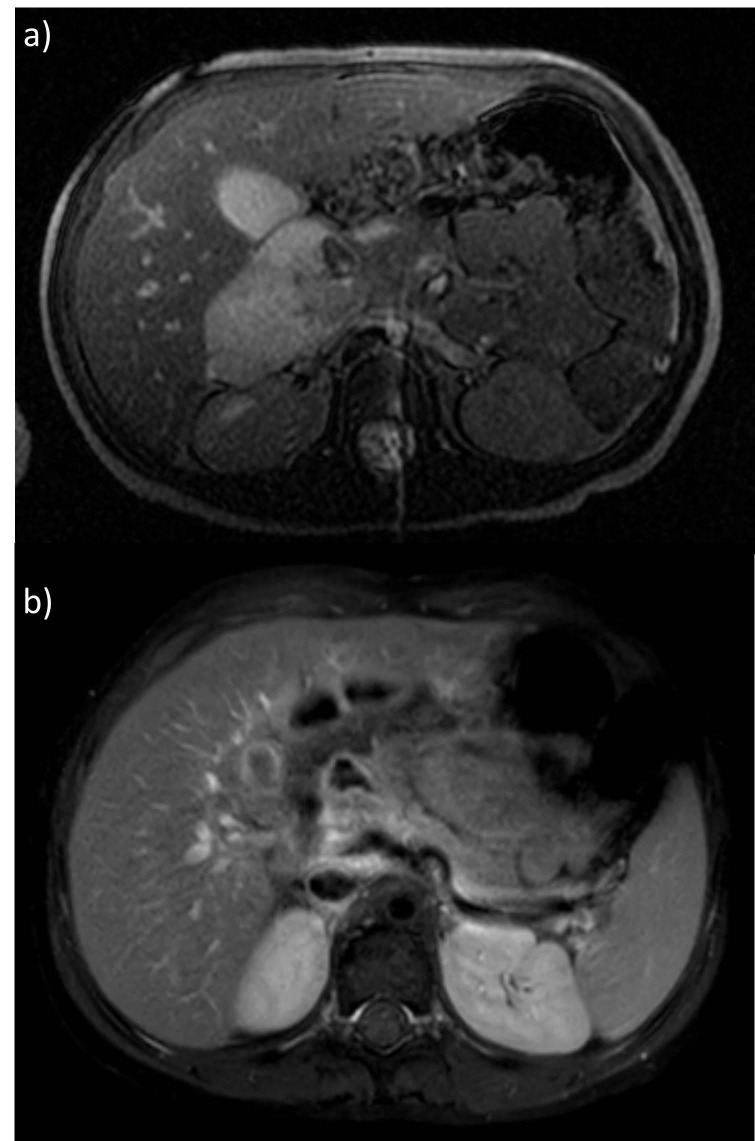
Magnetic resonance imaging (MRI) demonstrating significant response to chemotherapy and radiation. a) pre-treatment MRI, b) end-of-treatment MRI
